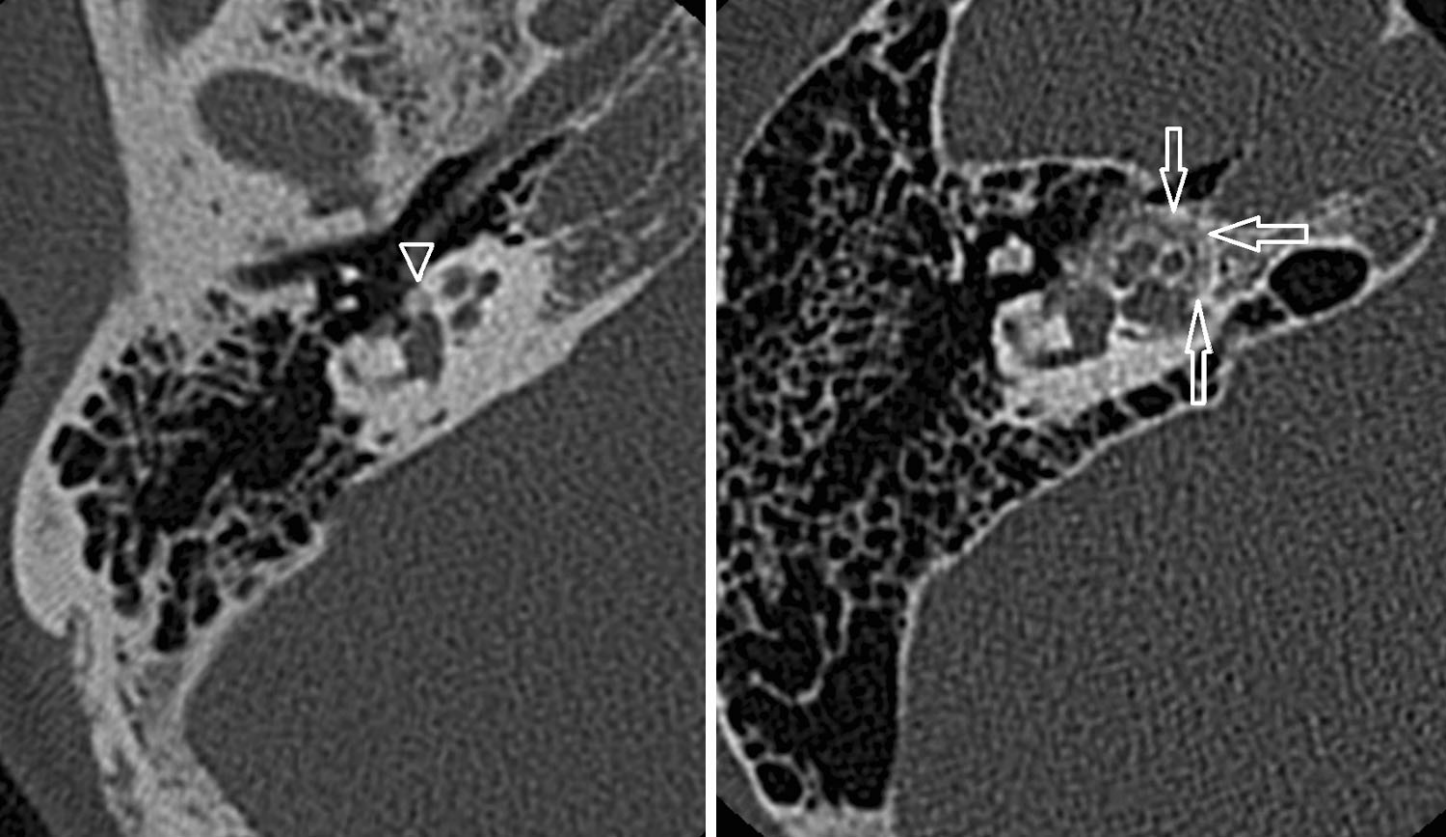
Fenestral (*left*) and cochlear otosclerosis (*right*) on axial thin-sliced CT. Lucency of the fissula antefenestram (*arrowhead*) in fenestral otosclerosis. Lucent halo surrounding the cochlea (*arrows*) in cochlear otosclerosis
